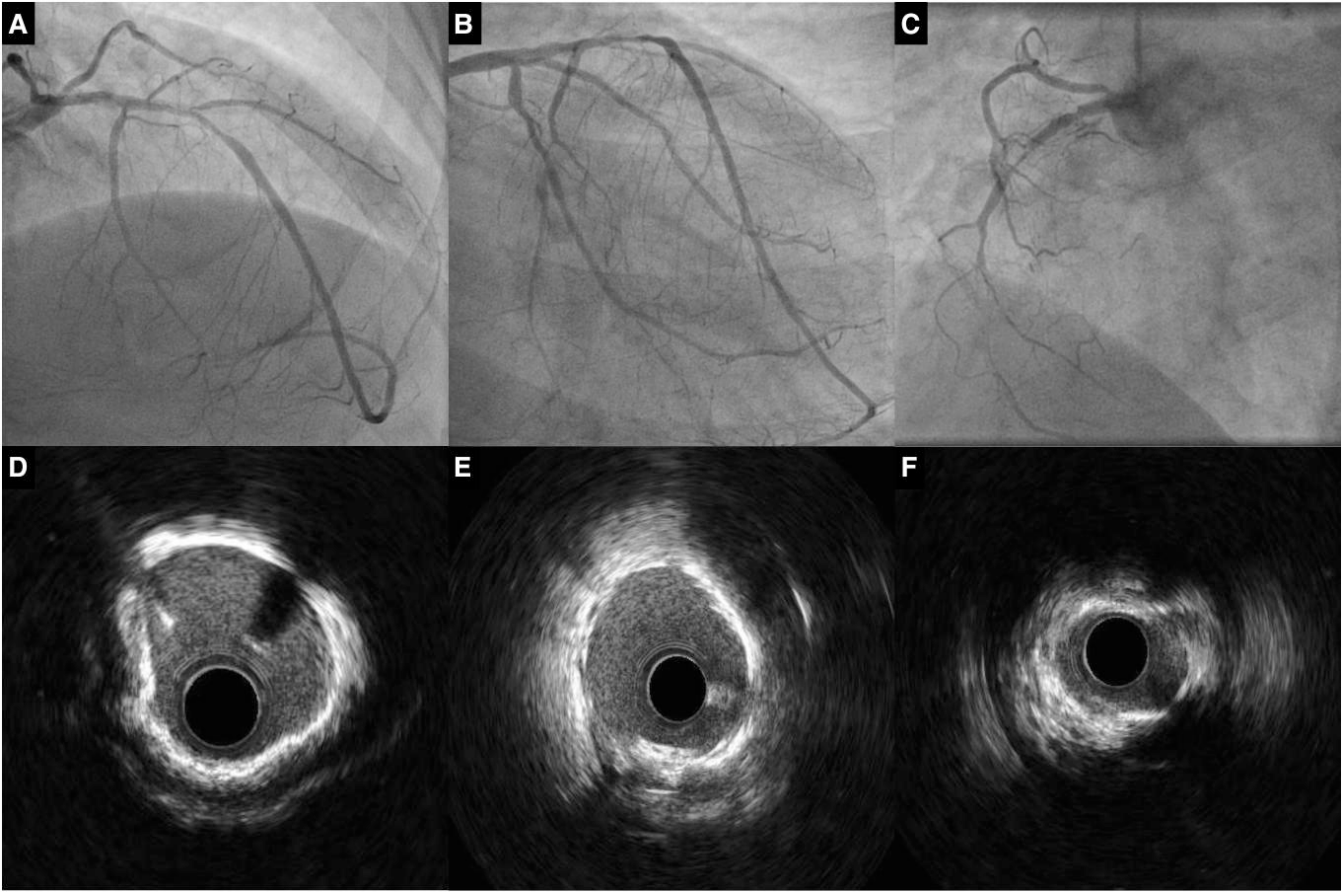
Coronary angiographies and intravascular ultrasound (IVUS) evaluation. (*A*) Cranial angiography showcasing left anterior descending (LAD) severe stenosis in the proximal and medium segment of the vessel. (*B*) Right caudal angiography showing left circumflex (LCx) severe stenosis in the proximal segment of the vessel. (*C*) Left oblique angiography displaying diffuse severe right coronary artery (RCA) stenosis. (*D*) IVUS of the LAD presenting a 360° calcification arch. (*E*) IVUS of the LCx revealing a 180° calcification arch. (*F*) IVUS of the RCA showing diffuse calcification and vessel sub-occlusion.
